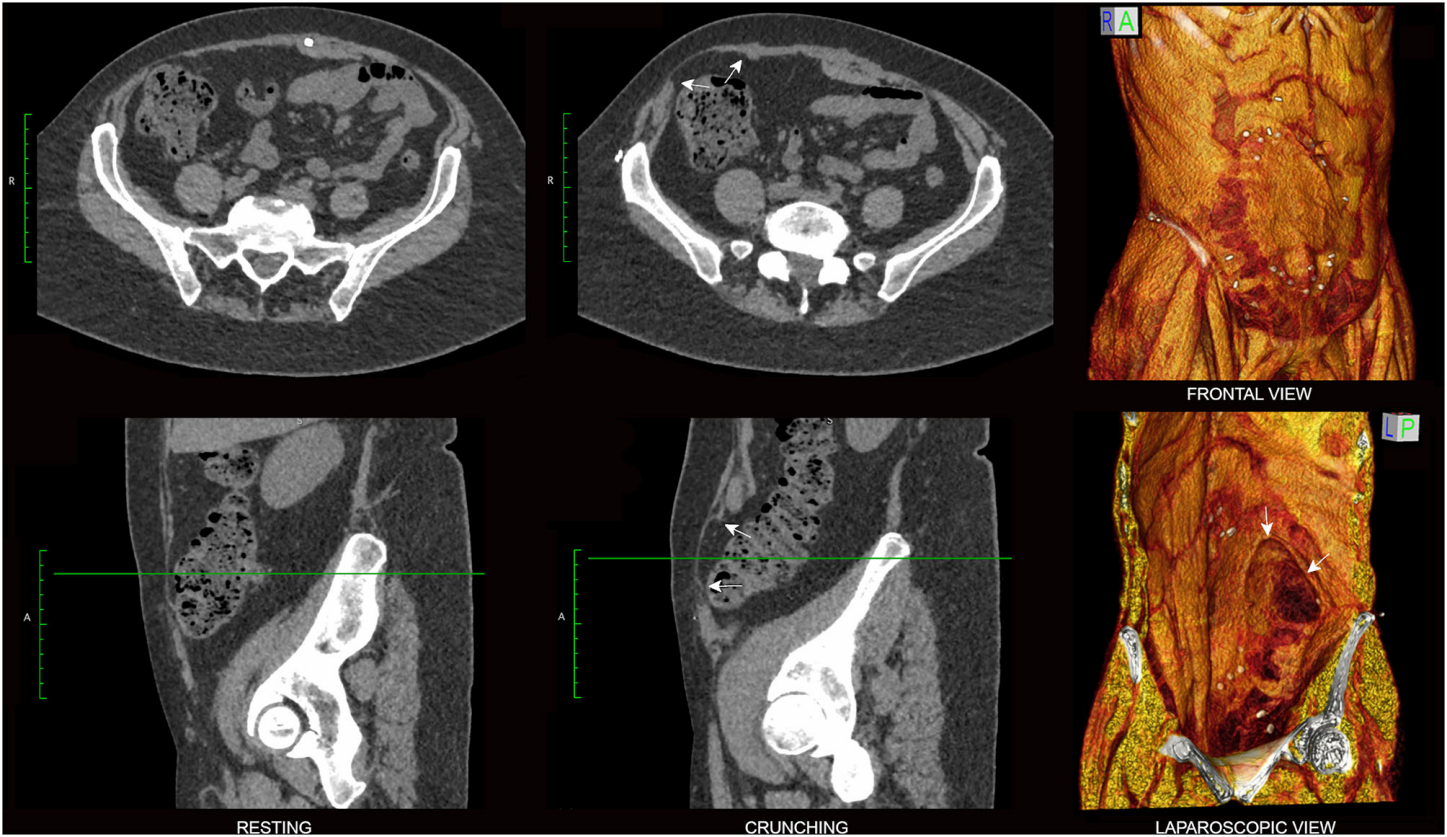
Hernia morphology. Resting (left) and crunching (center) phase axial (top) and sagittal (bottom) non-contrast CT images of a 58 year old female with right lower quadrant pain following TRAM flap procedure are shown with companion 3D volume rendered crunching images. In this case the differentiation of a dysfunctional bulge from shallow broad-based hernia was clinically uncertain, but the presence of abrupt ‘angular shouldering’ at the margins of the bulge (arrows) that was confidently visible only on the crunching phase CT images was regarded as diagnostic for hernia. Note mesh well-seen here as an ovoid non-anatomical “sheet-like” appearance overlying right RA muscle on the frontal view 3DVR image.
